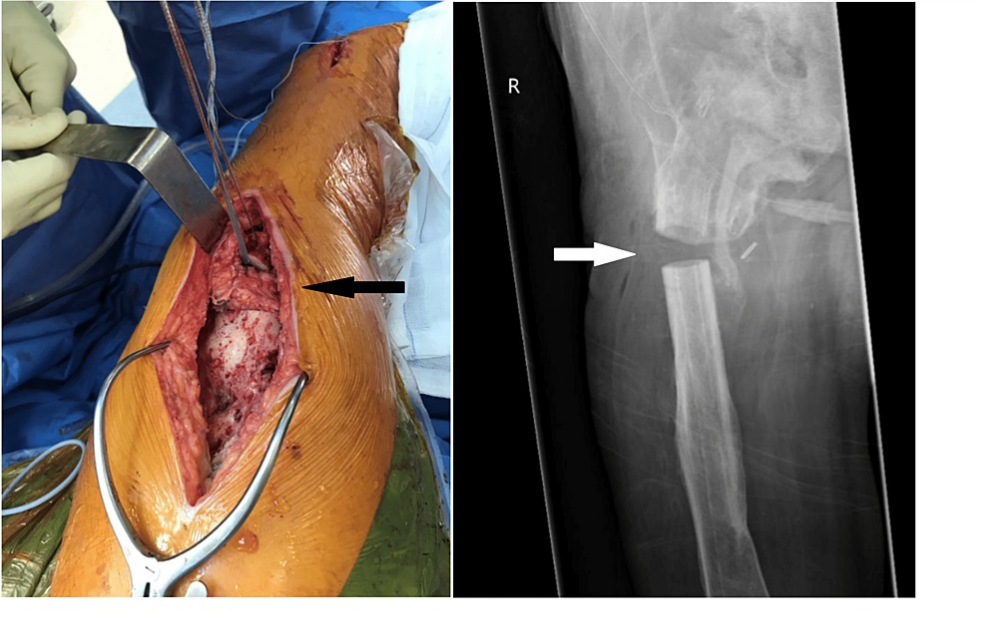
(Left) Intraoperative photograph showing the osteotomy, the vastus lateralis muscle flap interposition, and the transosseous fiber loop to prevent excessive posterior translation of the distal half of the femur (black arrow). (Right) Postoperative radiograph of the femur showing the partial femoral diaphysectomy (white arrow) with good alignment and remaining gap.
